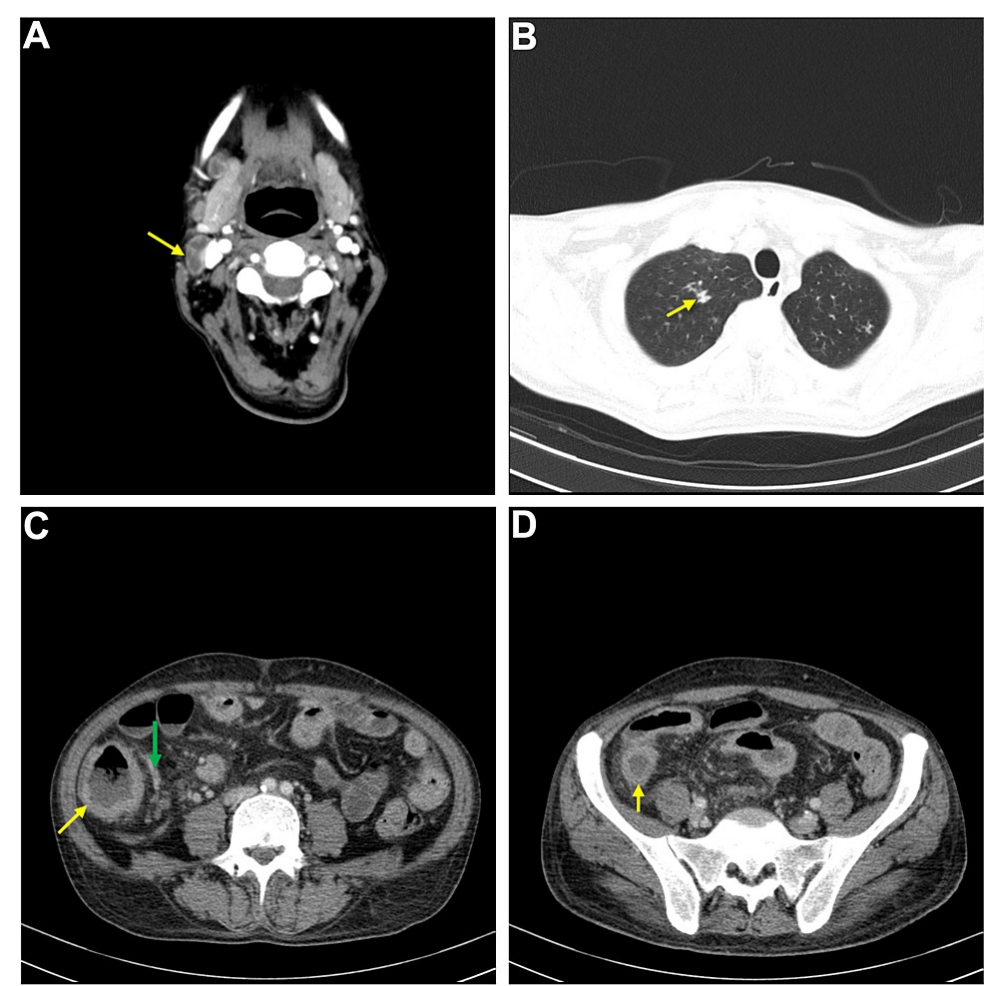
Contrast-enhanced cervical, thoracic, abdominal, and pelvic computed tomography. A: Jugular lymphadenopathy (yellow arrow); B: Small areas of fibrotic densification with traction bronchiectasis on the upper lobes (yellow arrow); C: Severe thickening of the ascending colon (yellow arrow) and adjacent mesentery (green arrow); D: Thickening of the cecum (yellow arrow).
